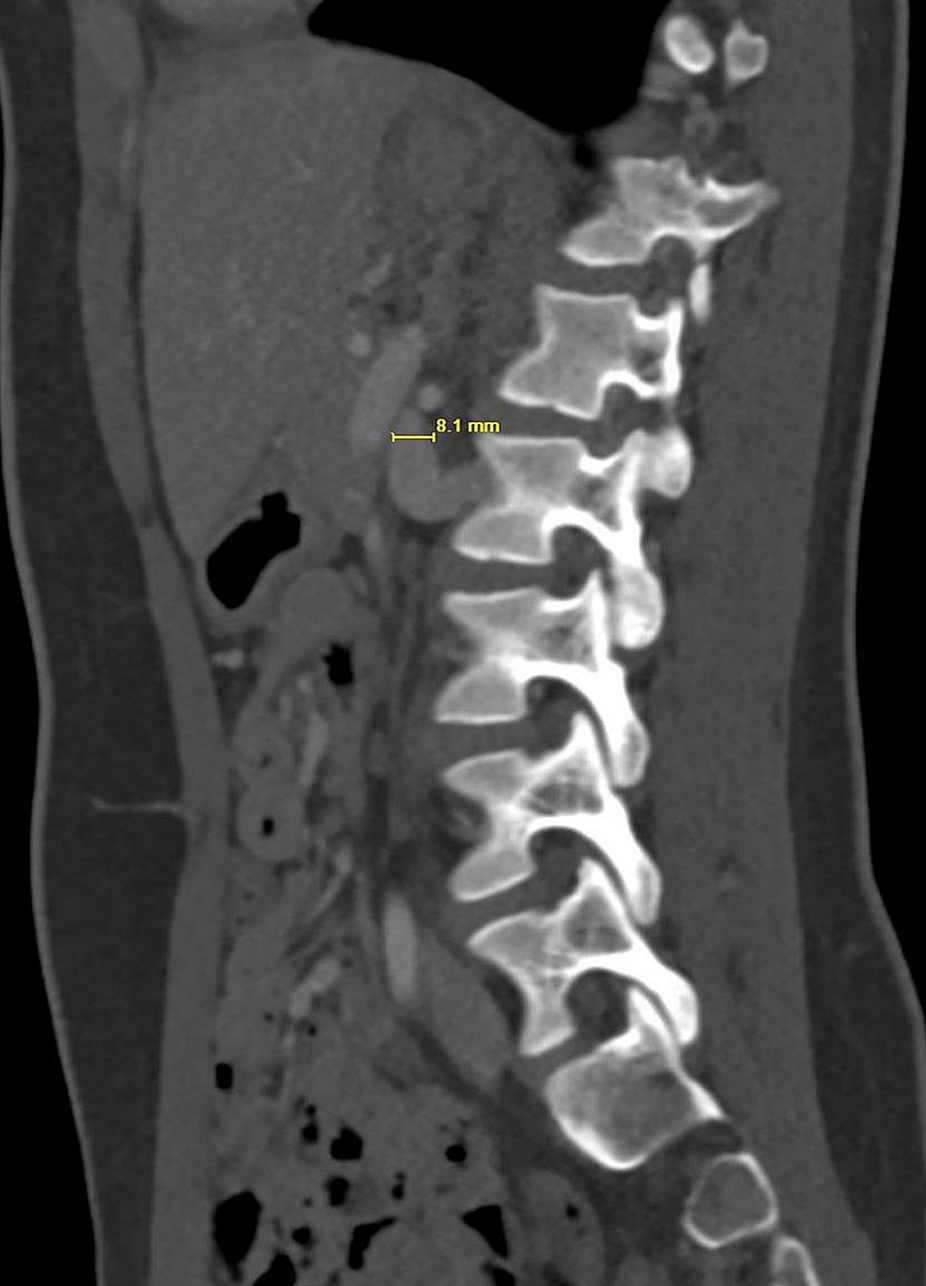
Left parasagittal CECT shows dilatation of the LRV proximal to the compression with an AP diameter of 8 mm. CECT - Contrast-enhanced computed tomography; AP - anteroposterior; LRV - left renal vein
